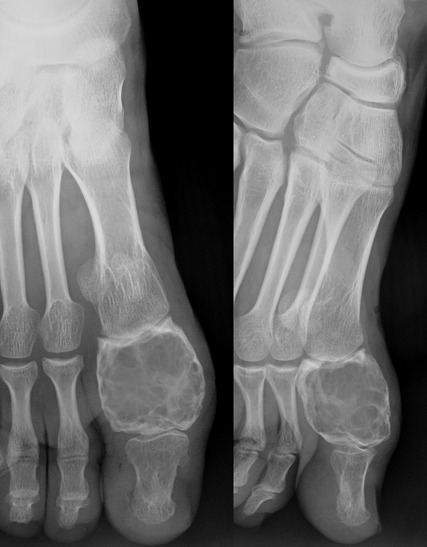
Anteroposterior and sagittal radiographs (5 year post operatively): expanding multiloculated osteolytic lesion involving the whole hallux phalanx
